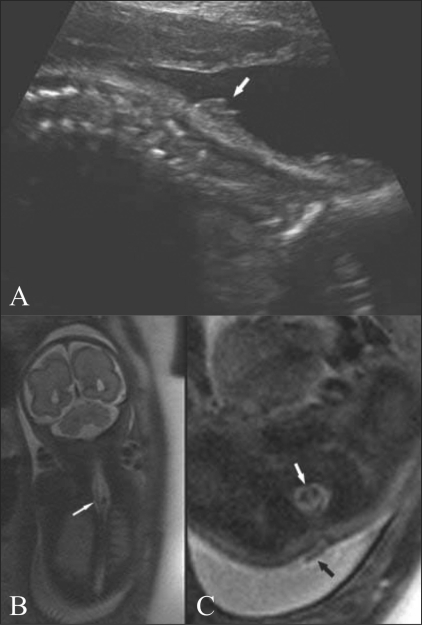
USG (A) of the fetal spine at the cervicodorsal level shows an abnormal soft tissue lesion over the skin (white arrow). Coronal (B) and axial (C), T2W HASTE MRI images show splitting of the cord (arrow in B), and a thick band (white arrow in C) separating the two cords. A band is seen over the skin surface (black arrow in C), with a track seen extending up to the spinal canal
